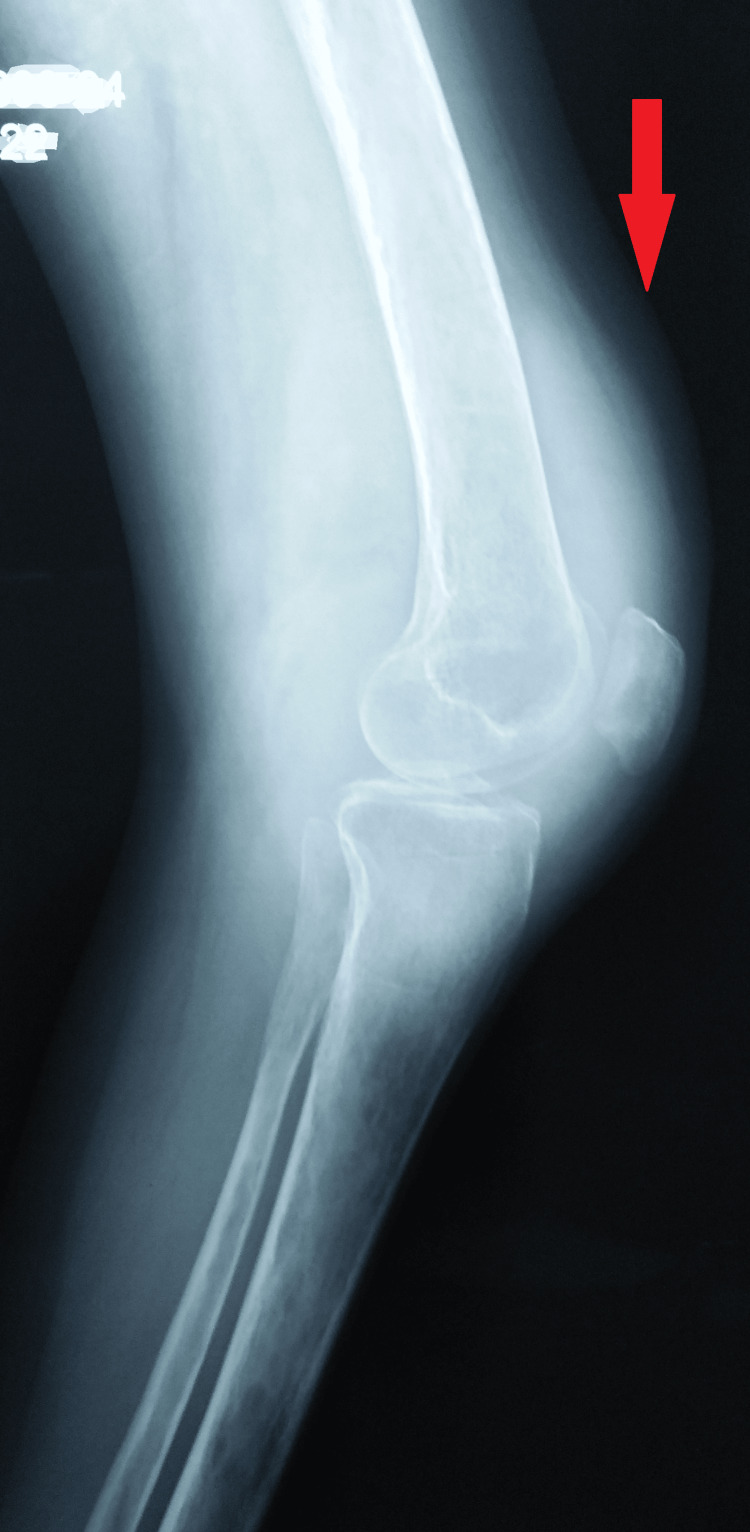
A plain radiograph of the swollen knee not suggestive of any erosion
